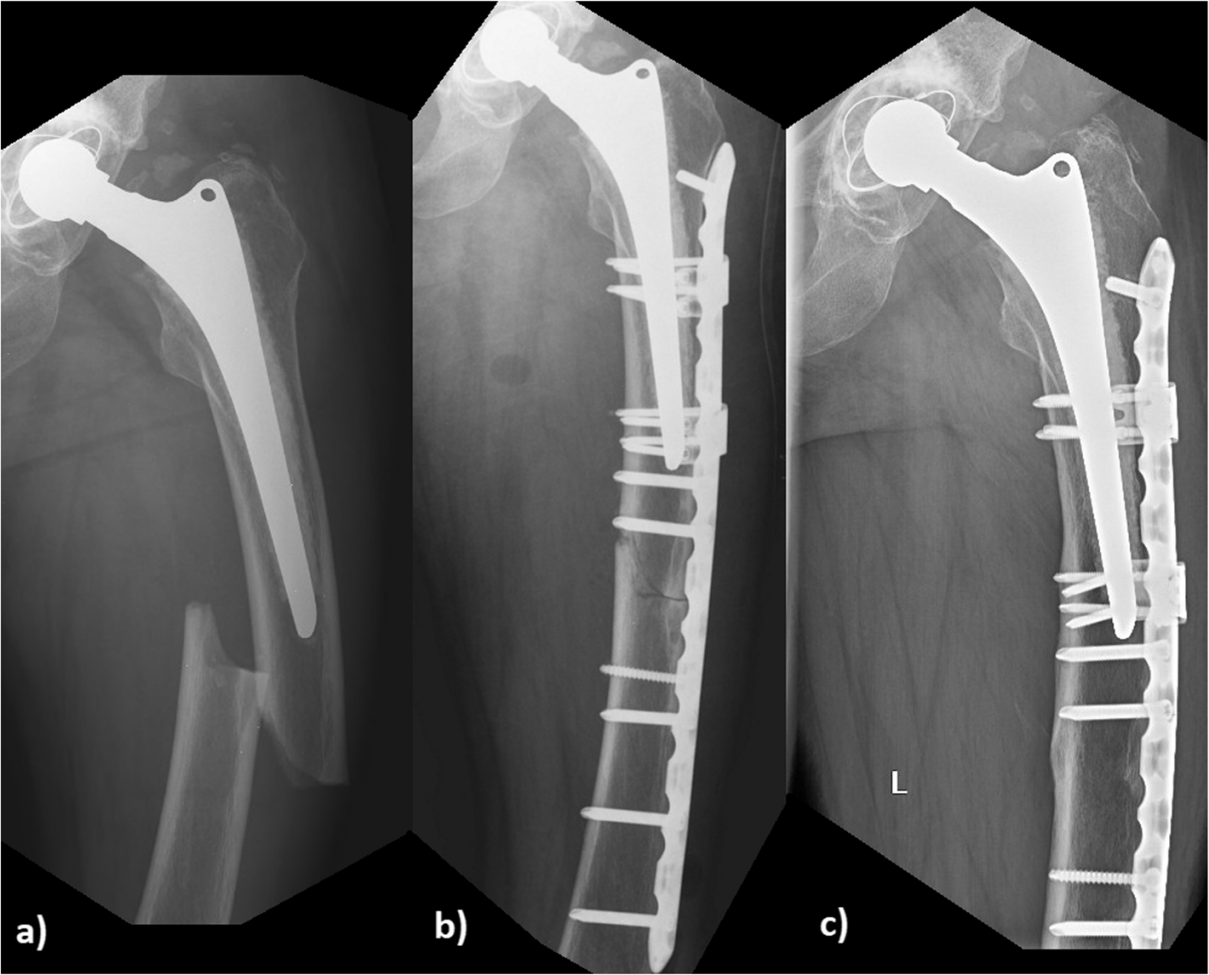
a 76-year-old female patient with an atypical periprosthetic femoral fracture, who took bisphofonates for 11 years. b Postoperative x-ray of the atypical fracture (operative fixation method with an 18 hole locking compression plate and 2 pieces of attachment). c X-ray of a healed atypical fracture after 1 year
